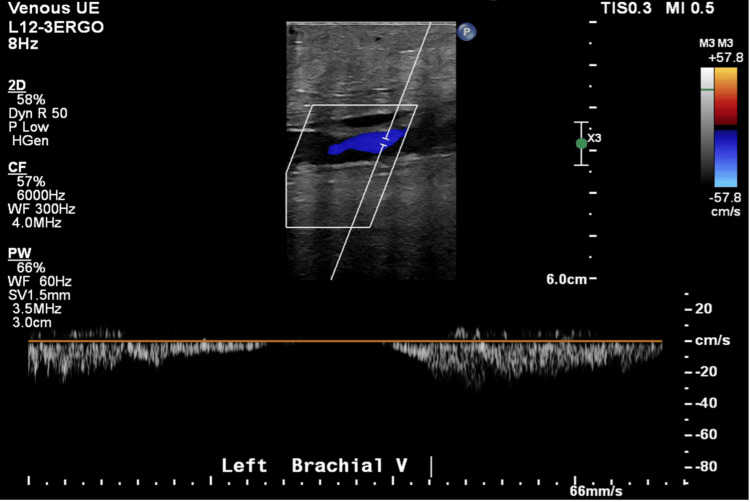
Left brachial vein duplex ultrasound.
